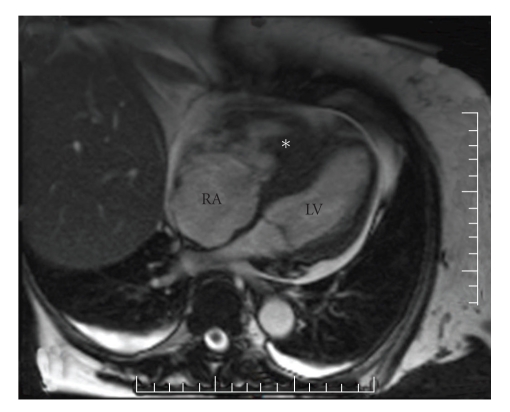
A magnetic resonance image (with gadolinium) shows a transverse view of the heart in diastole. The mass in the right ventricle (*asterisk*) fills most of the ventricle, and impedes filling of the left ventricle (LV). Note also the severe dilatation of the right atrium (RA) as well as the pericardial effusion (bright).
